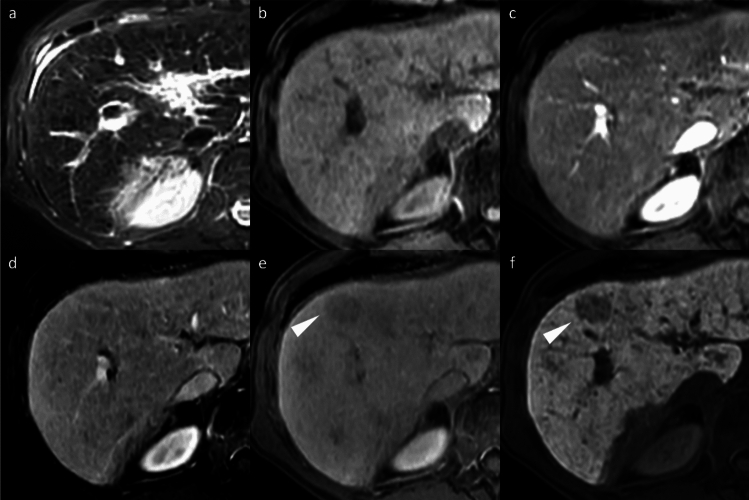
Hepatobiliary phase hypointense nodule without arterial phase hyperenhancement in a 69-year-old man with hepatitis B virus-related cirrhosis. A nodule (arrowhead) appears hypointense relative to the surrounding liver on the hepatobiliary phase image (f). It shows mild hypointensity on the transitional phase image (e; arrowhead), but no arterial phase hyperenhancement (c) or portal venous washout (d). The lesion is inconspicuous on fat-suppressed T2-weighted (a) and pre-contrast T1-weighted images (b)
